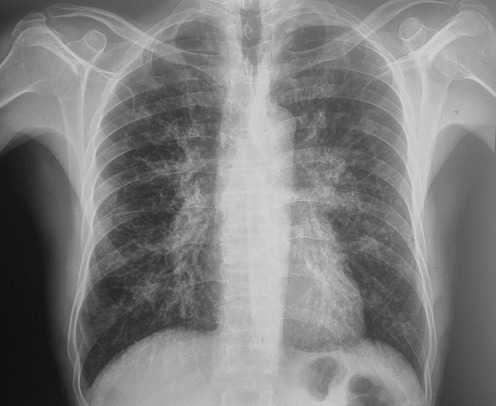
Radiographie standard du thorax
